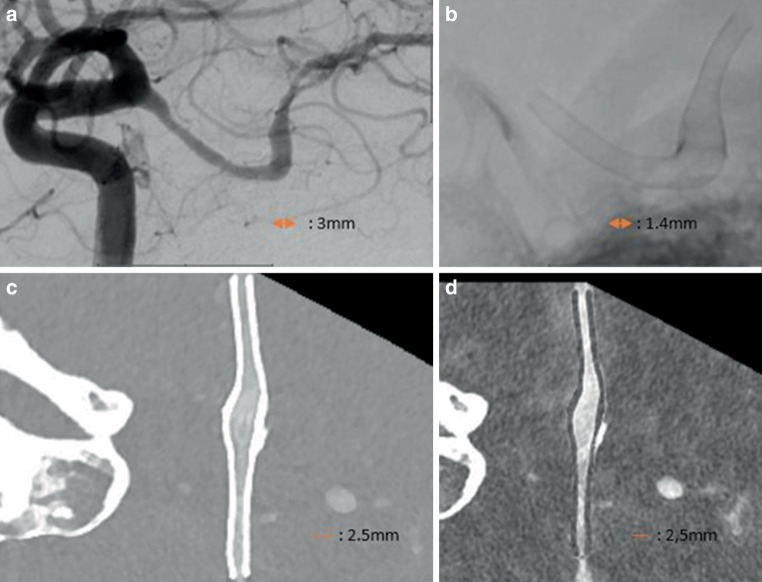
Two overlapping flow diverters in the right posterior communicating artery for the treatment of a fusiform aneurysm: a DSA lateral image, b blank image, radial reformations of the flow diverter of a VMI reconstruction c and iodine reconstruction d . Abbreviations: *DSA* Digital subtraction angiography, *PCD-CTA* Photon-Counting-Detector-CTA. *VMI* Virtual Monoenergetic Images
